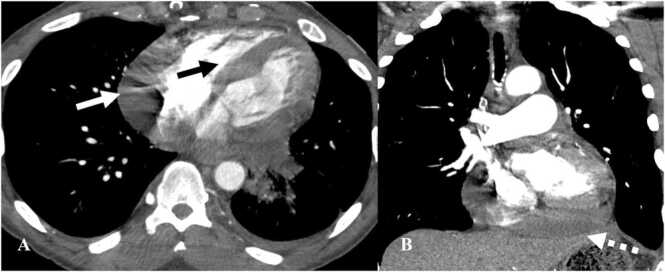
Immune-mediated pericarditis in a 67-year-old man with non-small cell lung cancer treated with Durvalumab. A. Contrast-enhanced axial chest CT image shows pericardial effusion along the right heart border (white arrow). Note the straightening of interventricular septum which can be seen with tamponade (black arrow). B. Coronal image in the same patient shows a large pericardial effusion at the base of the heart (dashed white arrow).
